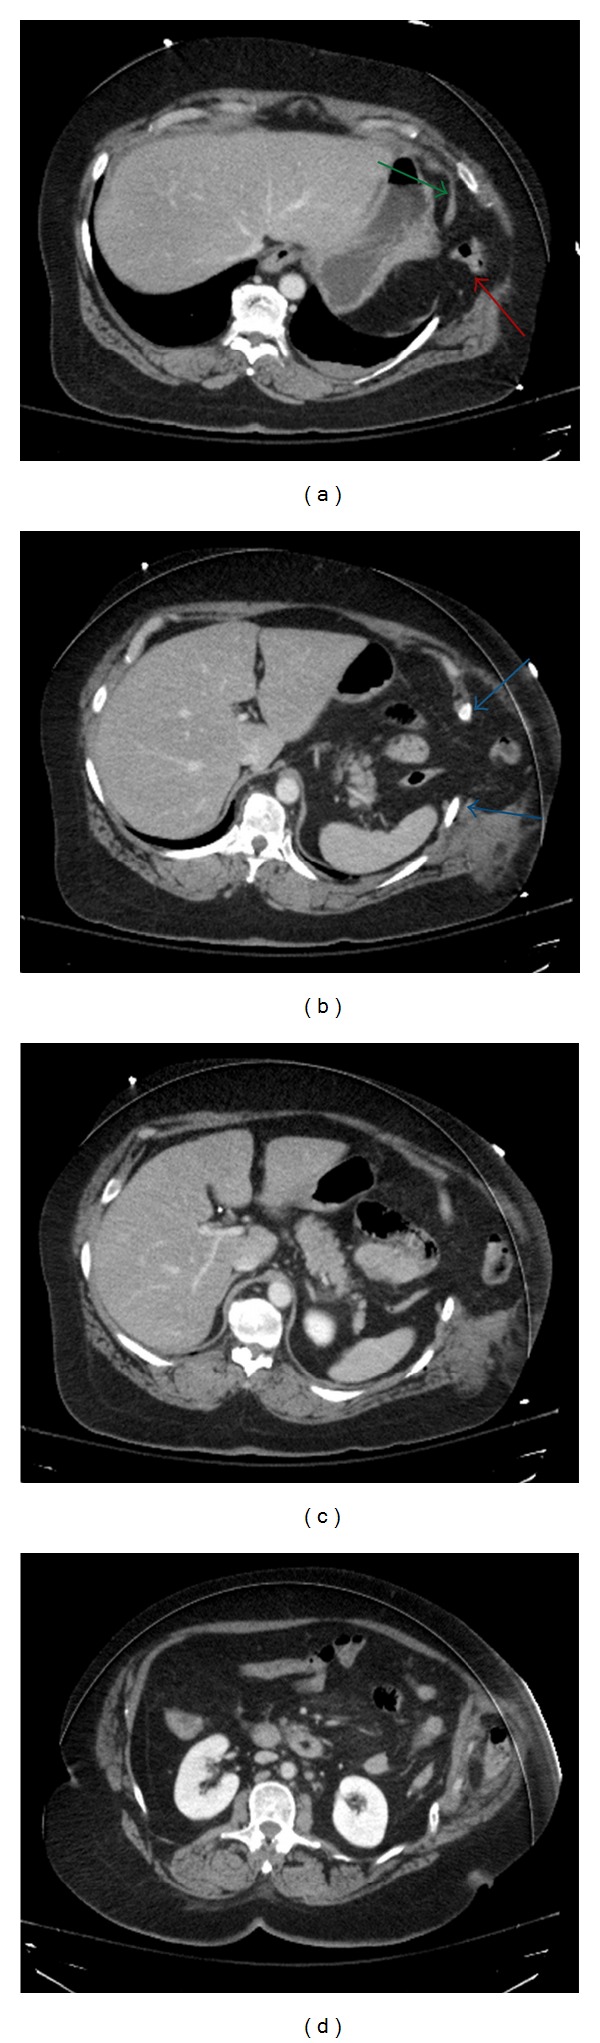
Axial CT images of the abdomen demonstrate herniation of abdominal contents is present including omentum and transverse colon into the chest and through the intercostal defect forming a hernia sac composed of peritoneum (red arrow) (a). There is discontinuity of the diaphragm (green arrows) and widened intercostal space (blue arrows).
